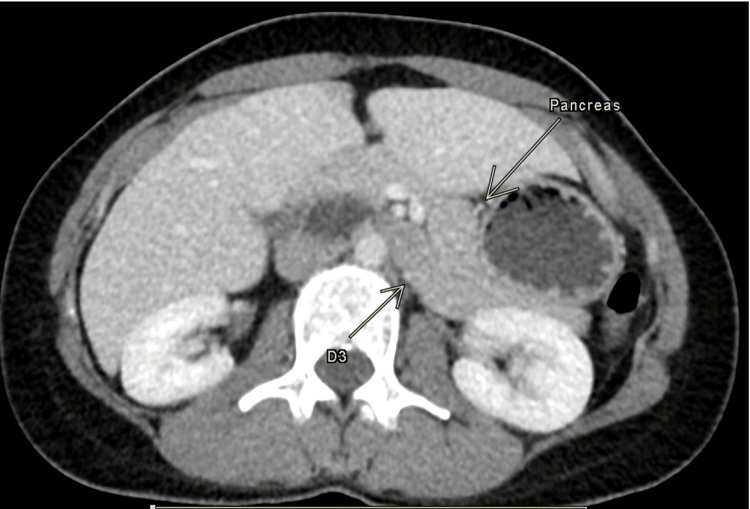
Post-contrast CT of the abdomen showing the third part of the duodenum (D3) at the level of the pancreatic body
